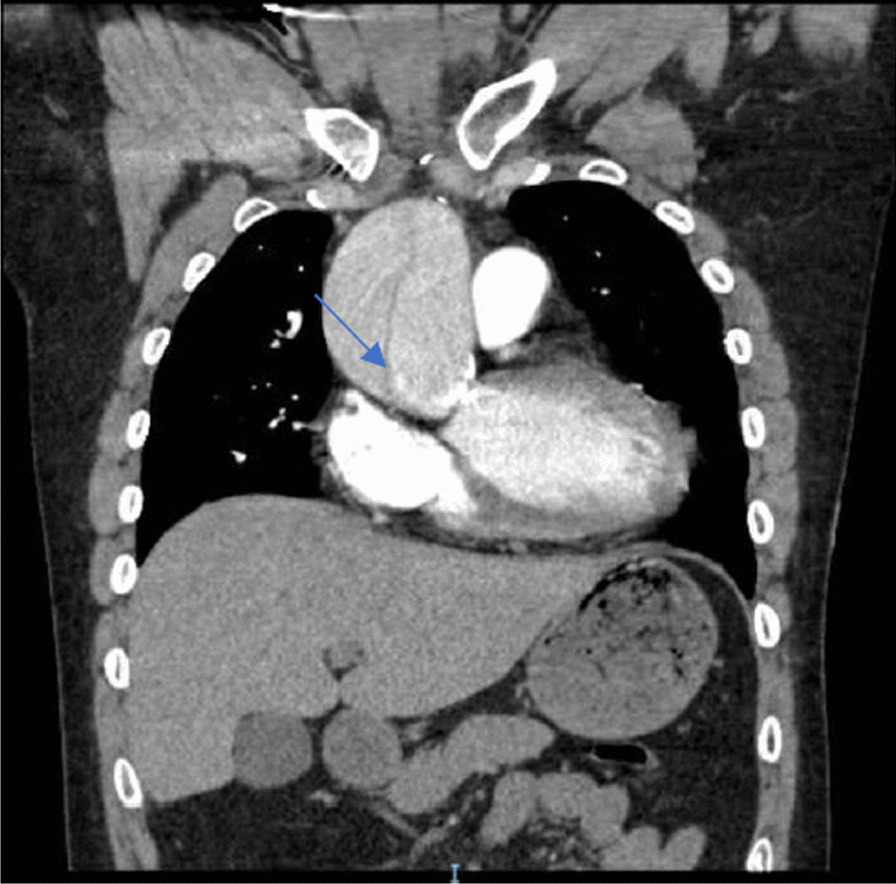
CTA chest coronal view demonstrating acute aortic dissection in setting of chronic aortic dilation (blue arrow)
